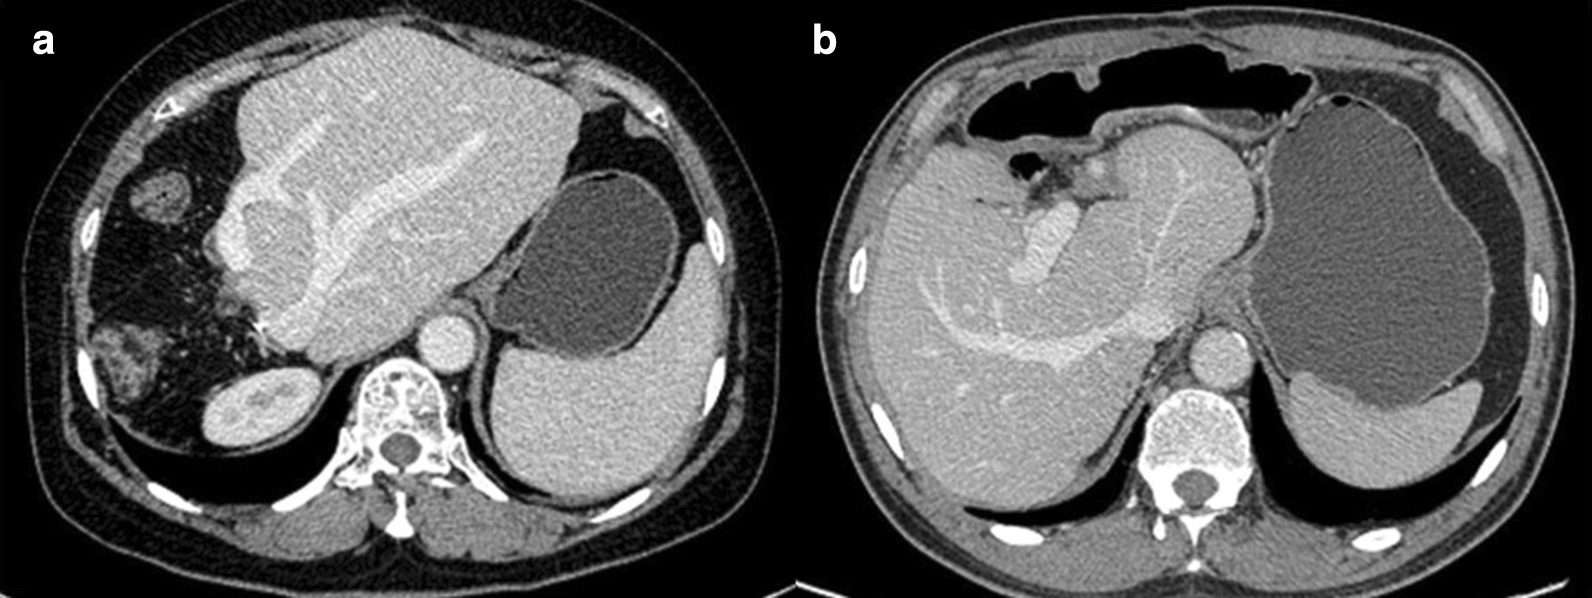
Follow-up CT image. No abnormality including stones, liver atrophy and bile duct dilatation were observed in the remanent liver of case 1 (a) and case 2 (b)
